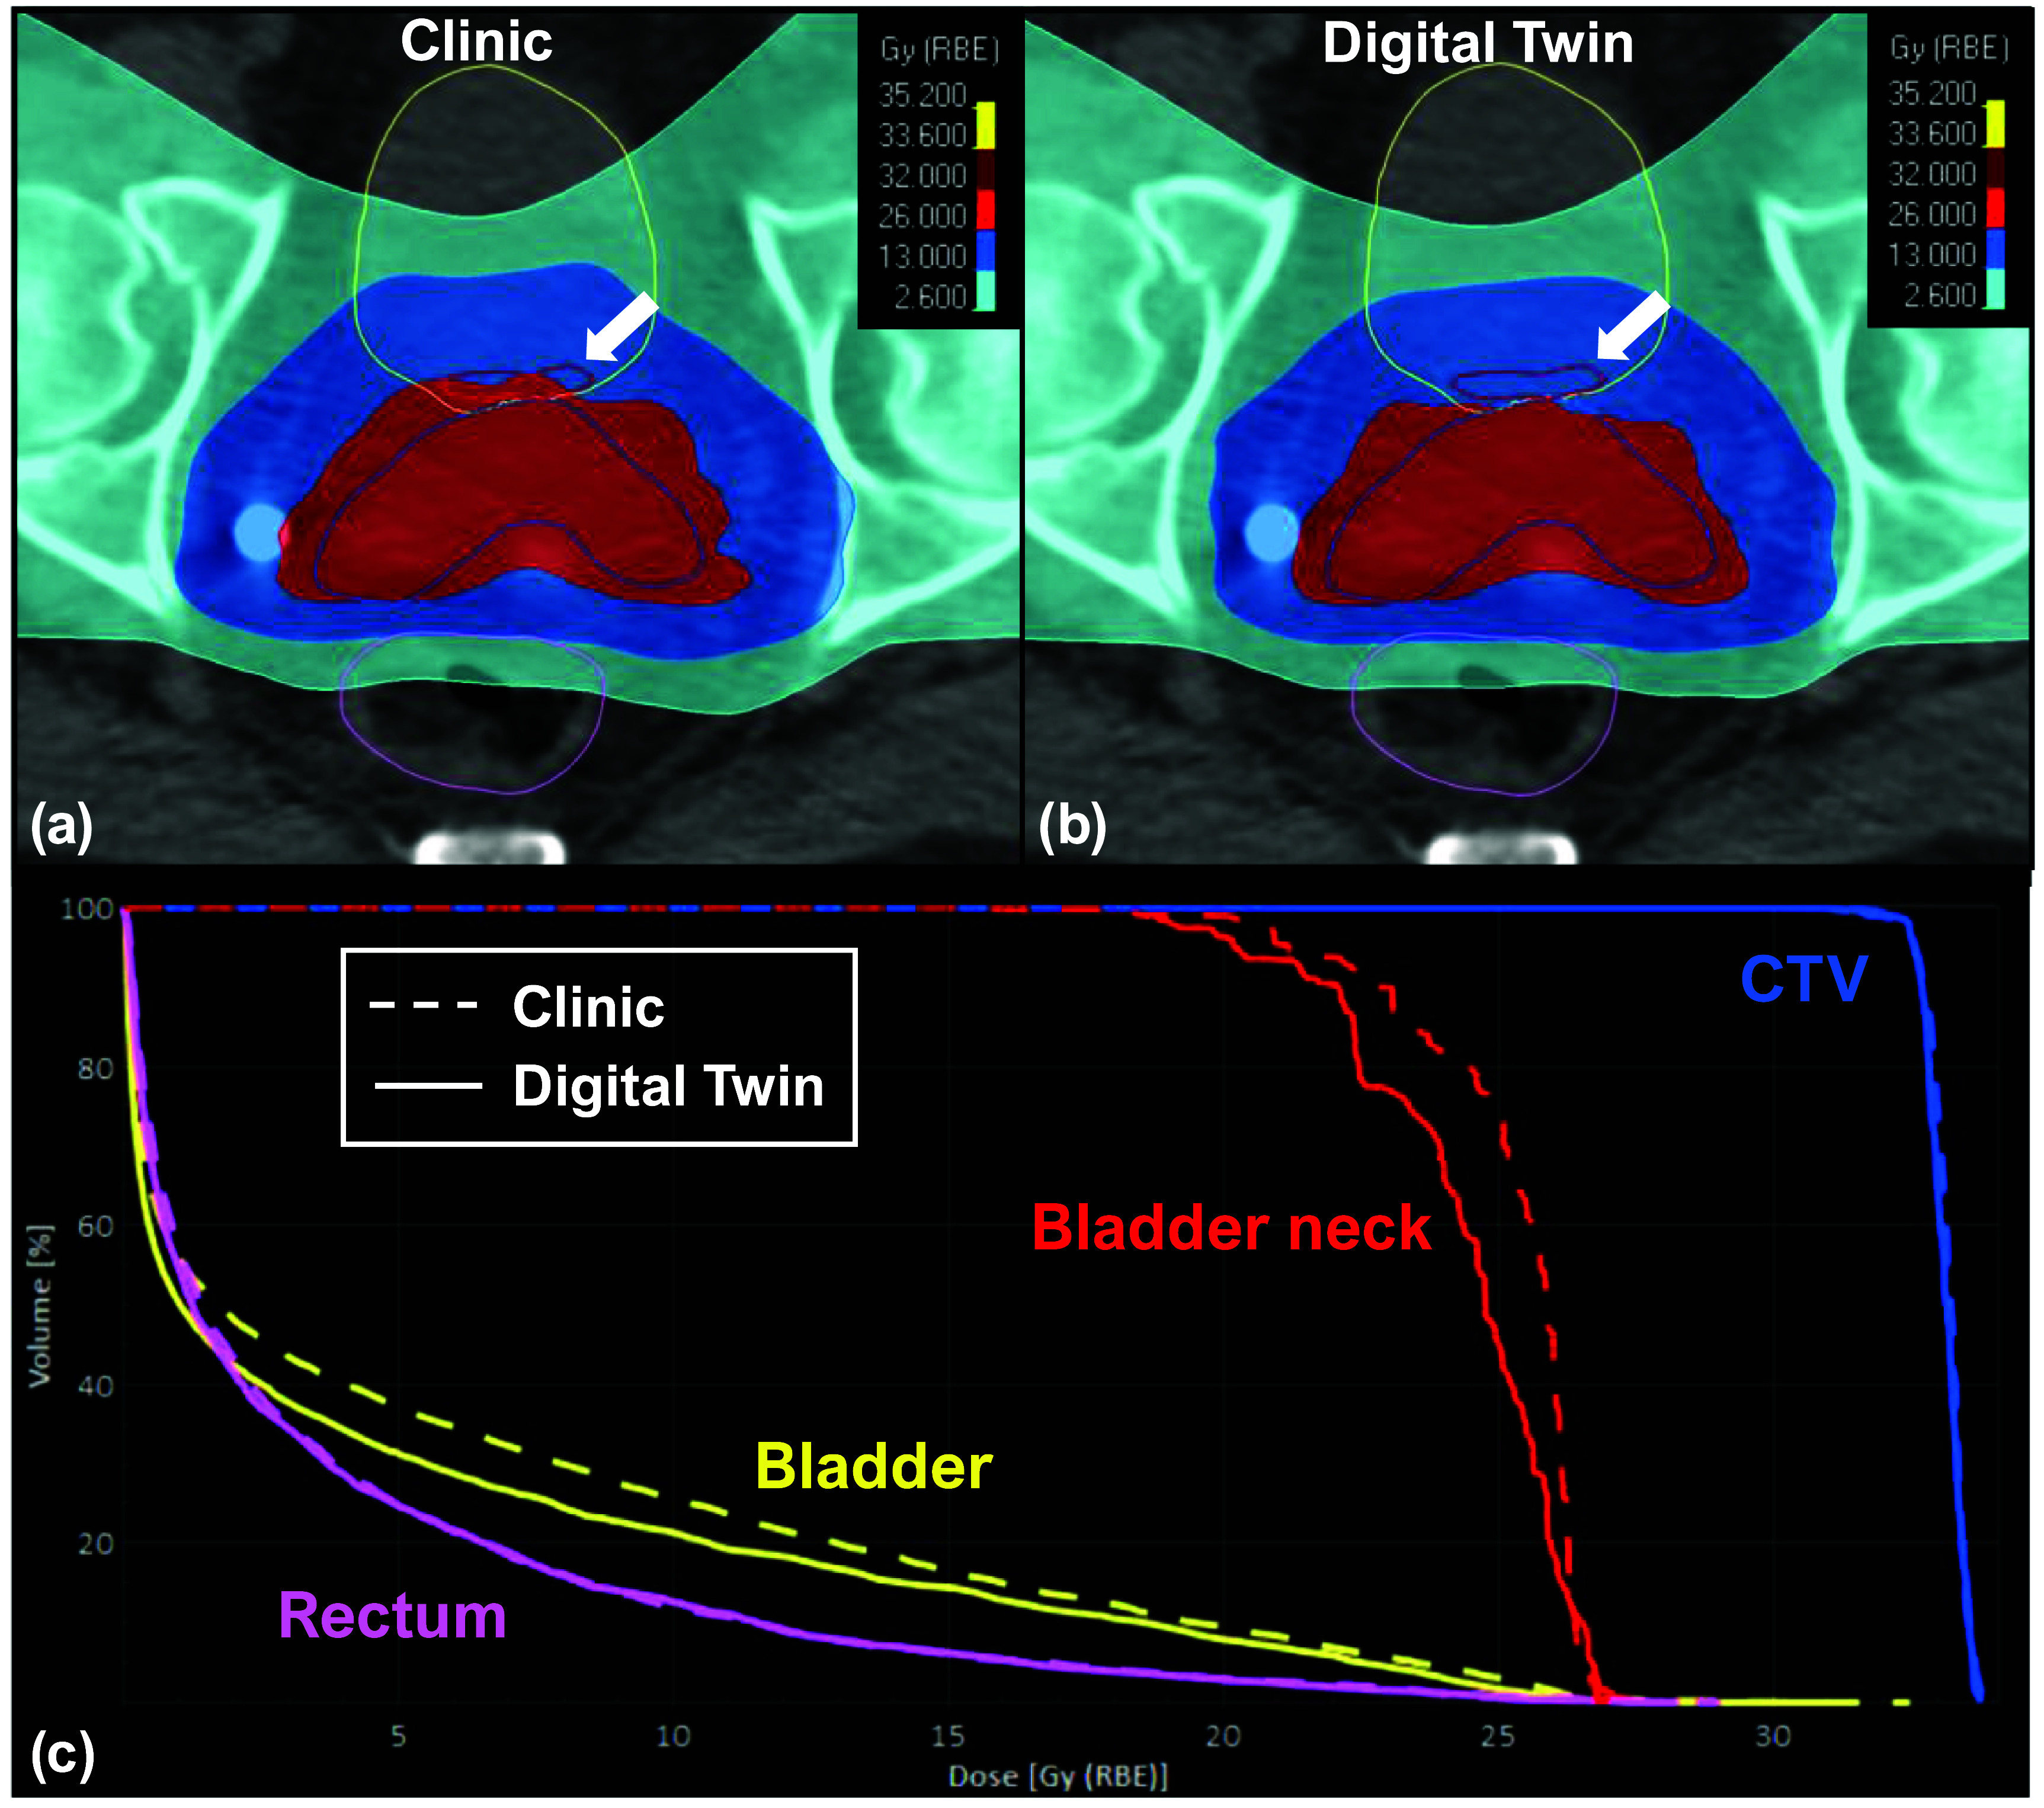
Dosimetry comparisons for Patient 8 between the clinical and DT-based plans. The top row displays the dose distributions in color wash for the (a) clinical plan and (b) DT-based plan, overlaid with transversal CT images and the contours of CTV (blue lines), bladder (yellow lines), bladder neck (red lines) and rectum (magenta lines). The bottom row (c) displays the dose-volume histogram (DVH) for CTV and OAR structures, where the dashed and solid lines represent the clinical plan and DT-based plan. The white arrow indicates that the clinical plan results in a significant portion of the bladder neck being covered by the full prescription dose.
